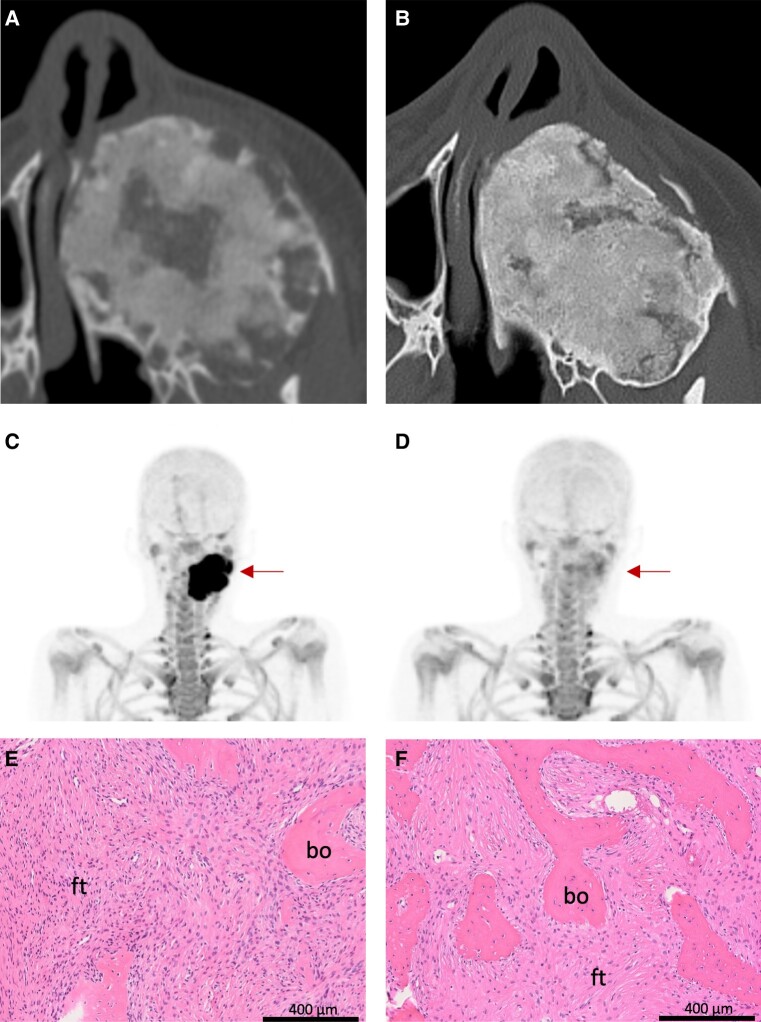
Representative images of denosumab treatment response in fibrous dysplasia (FD). The top panels show computed tomography images of a child with aggressive craniofacial FD. (A) Baseline scan at age 10 years shows an expansile lesion in the left maxilla. Note the heterogeneous appearance, with areas of radiolucency interspersed with more typically appearing “ground glass” radiopaque regions. (B) Two years after denosumab treatment the lesion has shown no further expansion and has developed a more homogenous, radiodense appearance. Based on her clinical response, the decision was made to discontinue denosumab treatment. The middle panels show ^18^F-NaF PET/CT imaging from this patient 3 years later (age 19 years), at which point FD expansion and pain had returned. (C) The maxillary lesion shows high tracer avidity (arrow), consistent with reactivation of FD lesion activity. The decision was made to restart denosumab treatment. (D) Six months later, the tracer avidity has declined to near-physiologic levels, correlating with improvement in her symptoms. The bottom panels show FD tissue from an adult patient pre- (E) and post- (F) denosumab treatment. Note the overall reduction in cellularity of the fibrous tissue (ft) and increased bone formation (bo) within the lesion.
